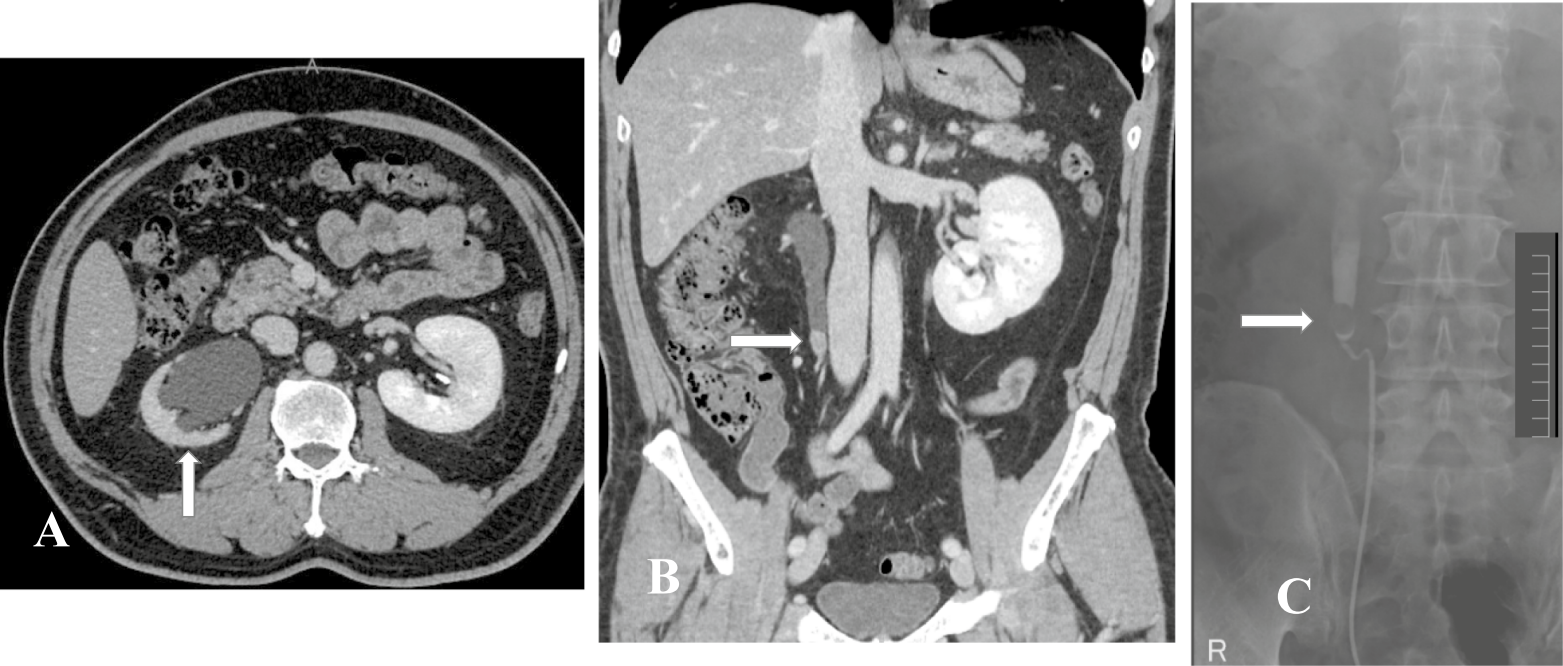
Abdominopelvic enhanced CT showing right ureter with obstruction, contracted kidney, and hydronephrosis (A: white arrow). Homogeneous enhanced tumor (B: white arrow). A filling defect of approximately 20-mm diameter in the upper right ureter is demonstrated by retrograde pyelogram (C: white arrow)
